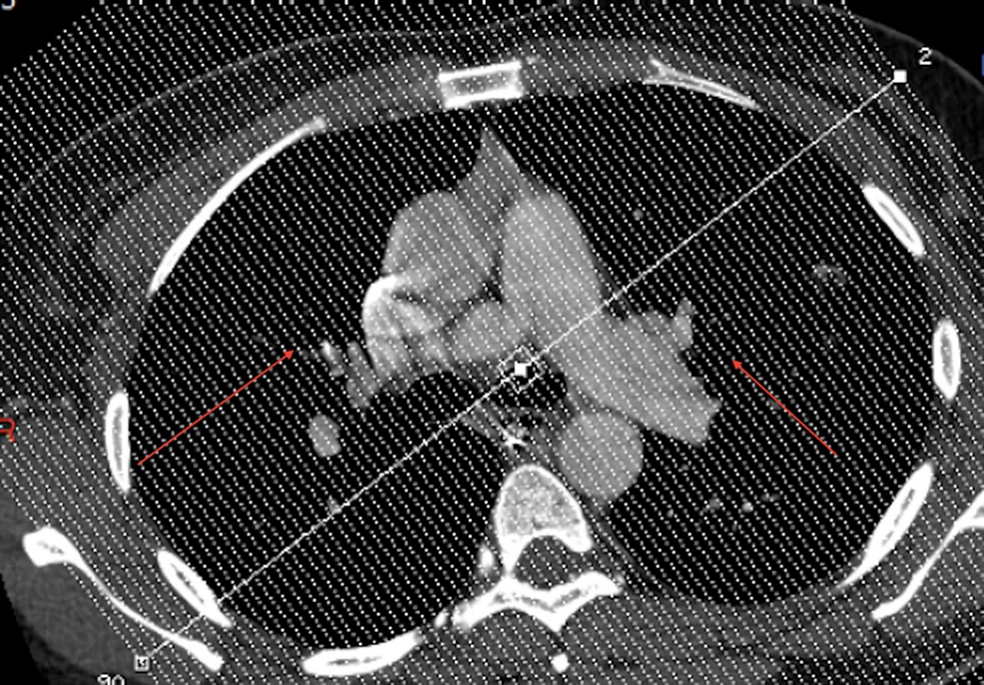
Computed tomography of the patient's chest indicating bilateral pulmonary infiltrates (red arrows)
